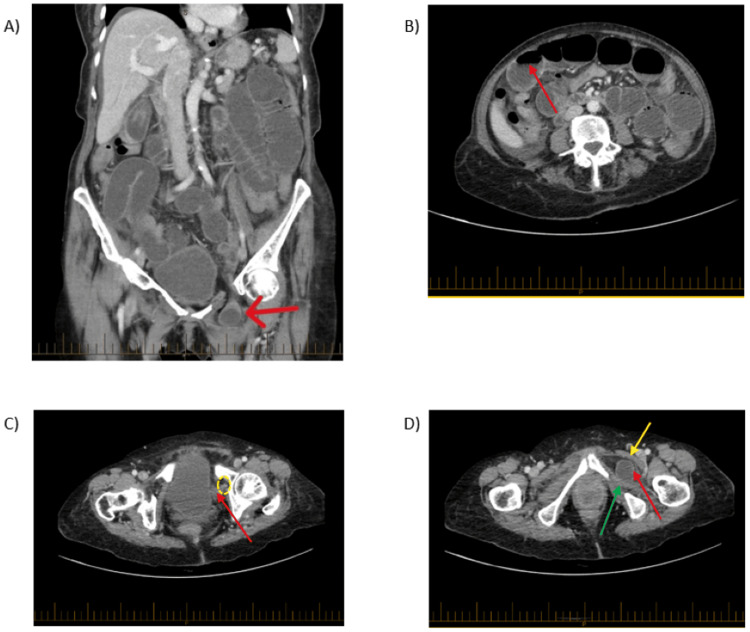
CT slice images of abdomen/pelvis with intravenous contrast in portal venous phase seen on window (W):369 level (L):40 level. A: CT coronal slice image of 5 mm slice thickness demonstrating small bowel obstruction (SBO) with dilatation of the small bowel with mesenteric stranding secondary to a left obturator hernia (red arrow) as the transition point where there is a protruding short segment of mid-ileum. There is a small volume of free fluid in the abdomen and pelvis, most notably surrounding the right lobe of the liver, with no evidence of portal venous gas or intra-abdominal free air. B: CT axial slice image of 3 mm slice thickness showing dilated loops of small bowel measuring up to 4 cm in diameter associated with air fluid levels (red arrow) with no evidence of abnormal mural thickening of bowel loops or pneumatosis intestinalis. C: CT axial slice image of 3 mm slice thickness showing an obturator canal (yellow dotted oval) and hernia protruding into the obturator canal (red arrow). D: CT axial slice image of 3 mm slice thickness showing loop of bowel (red arrow) between the pectineus (yellow arrow) and obturator externus (green arrow) [5].
